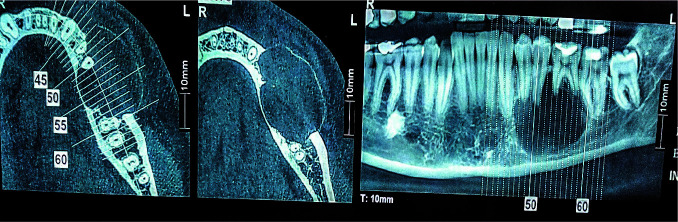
Cone beam computed tomography (CBCT) scans revealed a well-defined corticated unilocular radiolucent lesion of 34-37
